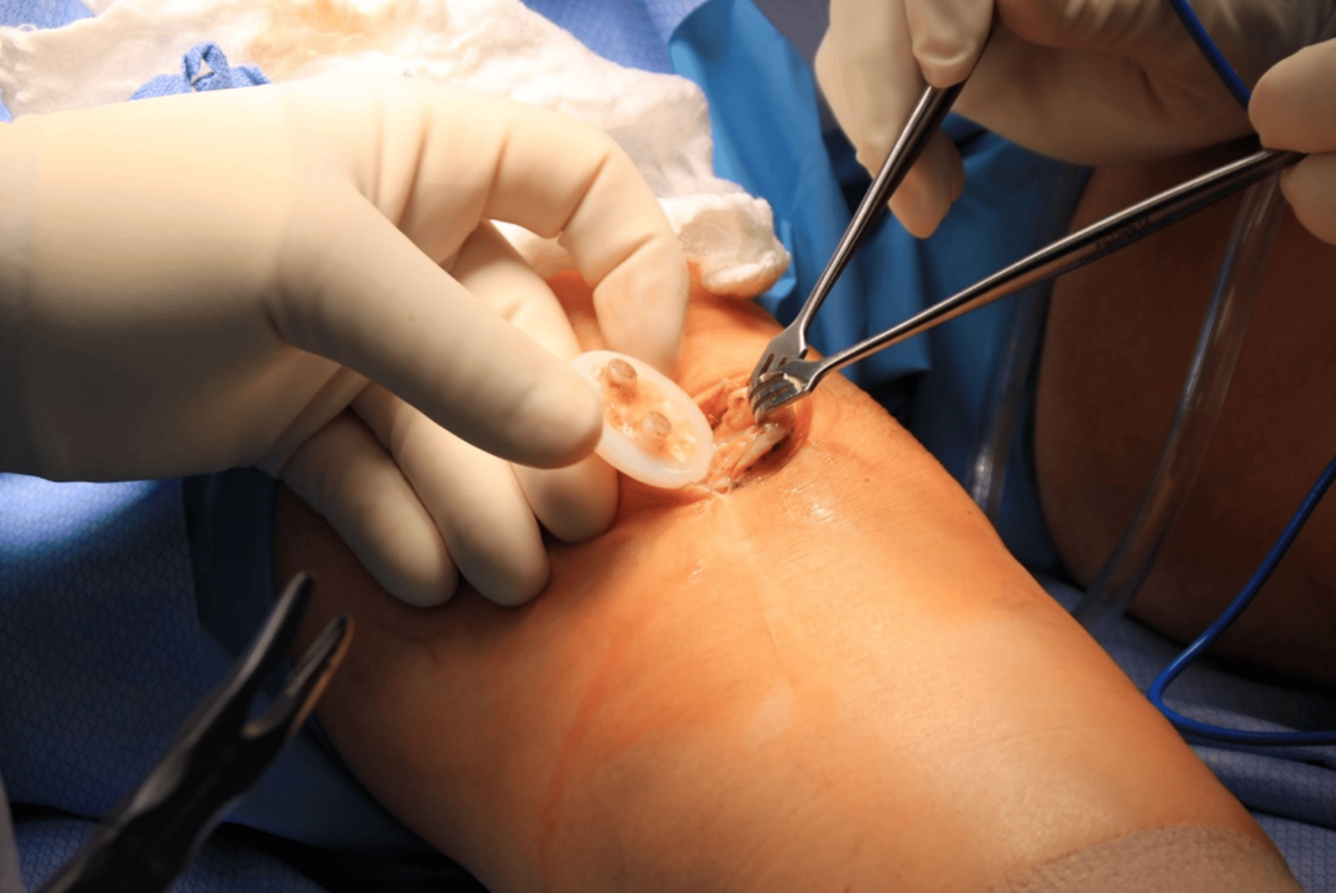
Excision of the patellar component demonstrating lack of native bone, confirming patella resorption seen on radiographs
